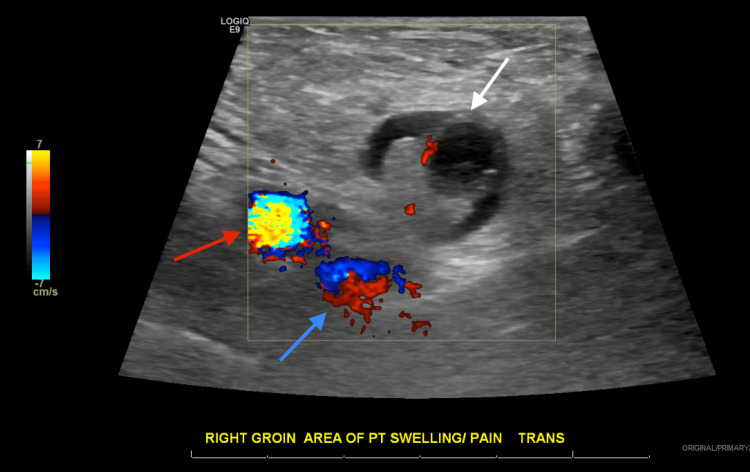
Ultrasound of the right groin Transverse view with color Doppler showing the femoral artery (red solid arrow) and vein (blue solid arrow) in relation to the hernia (white solid arrow).
